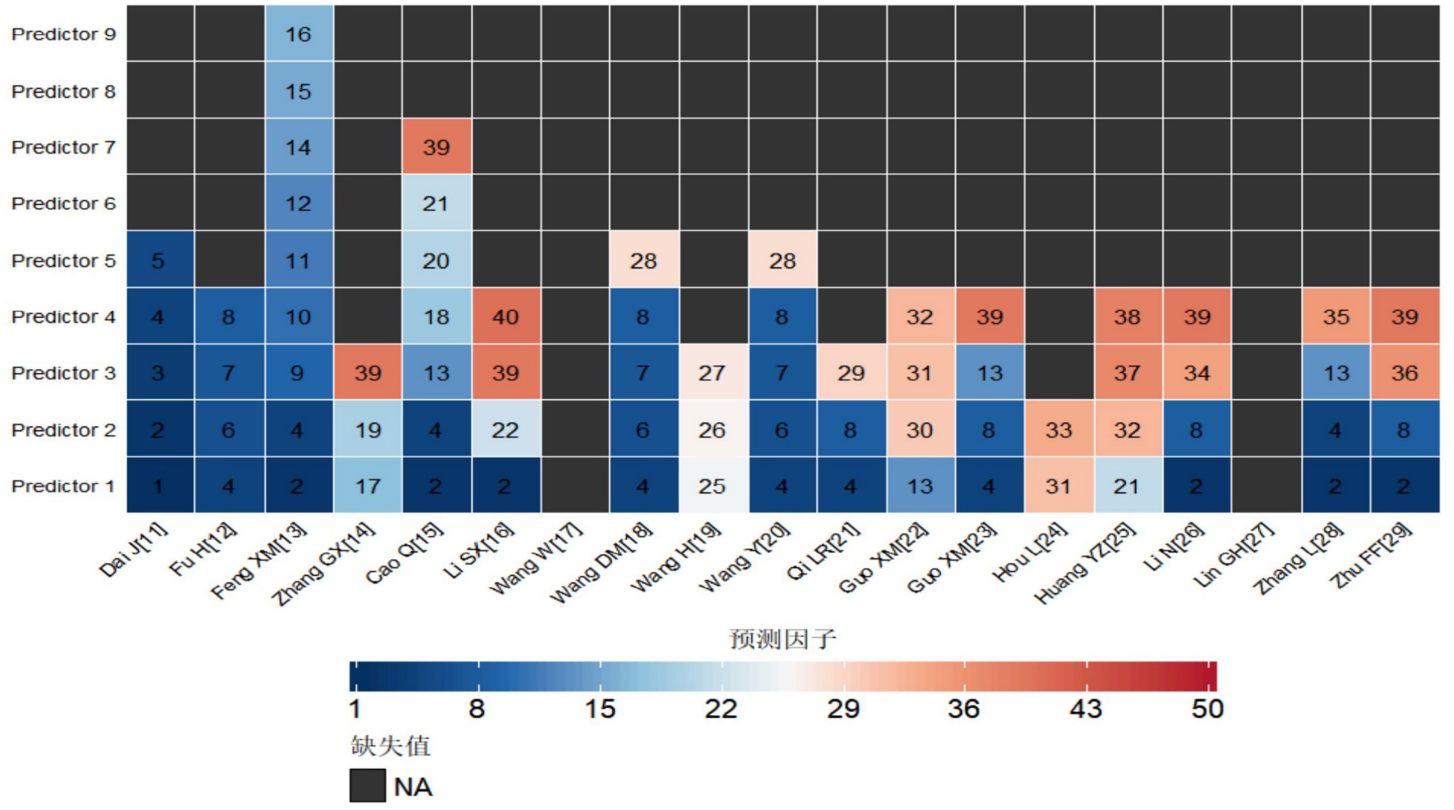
Predictors included in the prediction. 1, C-reactive protein (CRP); 2, Hypertension (HTN); 3, Carotid atherosclerosis; 4, Hyperhomocysteinemia (HHcy); 5, Degree of cerebral white matter lesions (WML); 6, Cystatin C (CysC); 7, Triglyceride-glucose index (TyG index); 8, High-load cerebral small vessel disease (CSVD); 9, Alcohol consumption; 10, Exercise duration <15 h; 11, Family history of dementia; 12, National Institutes of Health Stroke Scale score (NIHSS score); 13, Educational level; 14, Frontal lobe infarction; 15, Thalamic infarction; 16, Basal ganglia infarction; 17, Assessment of intracranial arterial stenosis; 18, Sleep disorder (SD); 19, Severity grading and location of leukoaraiosis (LA); 20, Living alone; 21, Diabetes mellitus (DM); 22, Number of lacunar infarcts ≥1; 23, Total cholesterol (TC); 24, Low-density lipoprotein cholesterol (LDL-C); 25, Sum of lacunar infarction and lacunar lesion counts (LI); 26, Fazekas scale (Fazekas 评分); 27, Giant cell arteritis score (GCA); 28, Apolipoprotein A (ApoA); 29, Lipoprotein-associated phospholipase A2 (LP-PLA2); 30, Estimated glomerular filtration rate (eGFR); 31, Neutrophil-to-lymphocyte ratio (NLR); 32, Male gender; 33, Fasting blood glucose (FBG); 34, Apolipoprotein A1 (ApoA1); 35, Total score of cerebral small vessel disease on MRI (CSVD MRI total score); 36, Lacunar infarction score; 37, Insulin-like growth factor 1 (IGF-1); 38, White matter lesions (WML); 39, Age; 40, High-density lipoprotein cholesterol (HDL-C).
